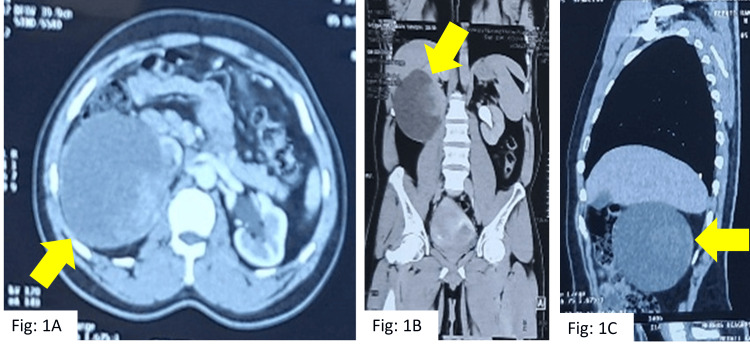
Contrast-enhanced computed tomography (CECT) showing right kidney lower pole cystic renal mass (10 * 11 * 12 cm) with solid component (5 * 5.4 * 4.2 cm). (A) A cystic mass with a solid component is seen in axial CECT (yellow arrow). (B) A large cystic mass can be seen below the liver (arrow), almost occupying the whole kidney. The normal kidney is on the opposite side. (C) Same cystic mass in the sagittal section.
